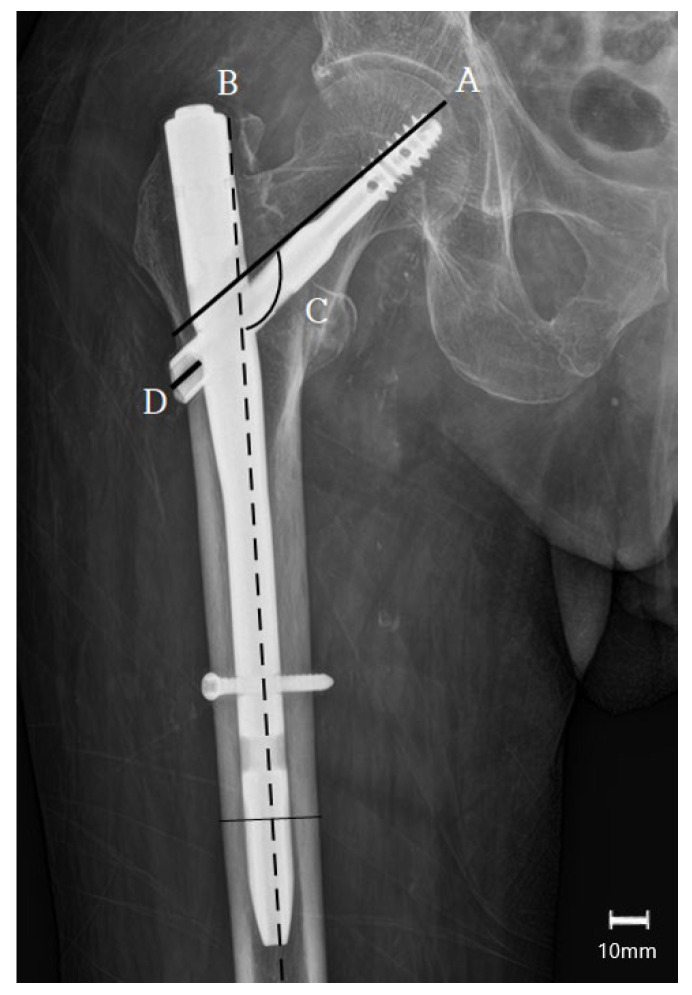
Measurement of lag screw sliding and varus collapse (ΔNSA) on anteroposterior radiographs. A: femoral neck axis; B: femoral shaft axis; C: neck–shaft angle (NSA), an angle formed by line A and B. Varus collapse is the change in NSA (angle C) between time points. Lag screw sliding is defined as the change in distance D, measured between the immediate postoperative and follow-up periods (angles are reported in degrees (°) and distances in millimeters (mm). Scale bar = 10 mm).
